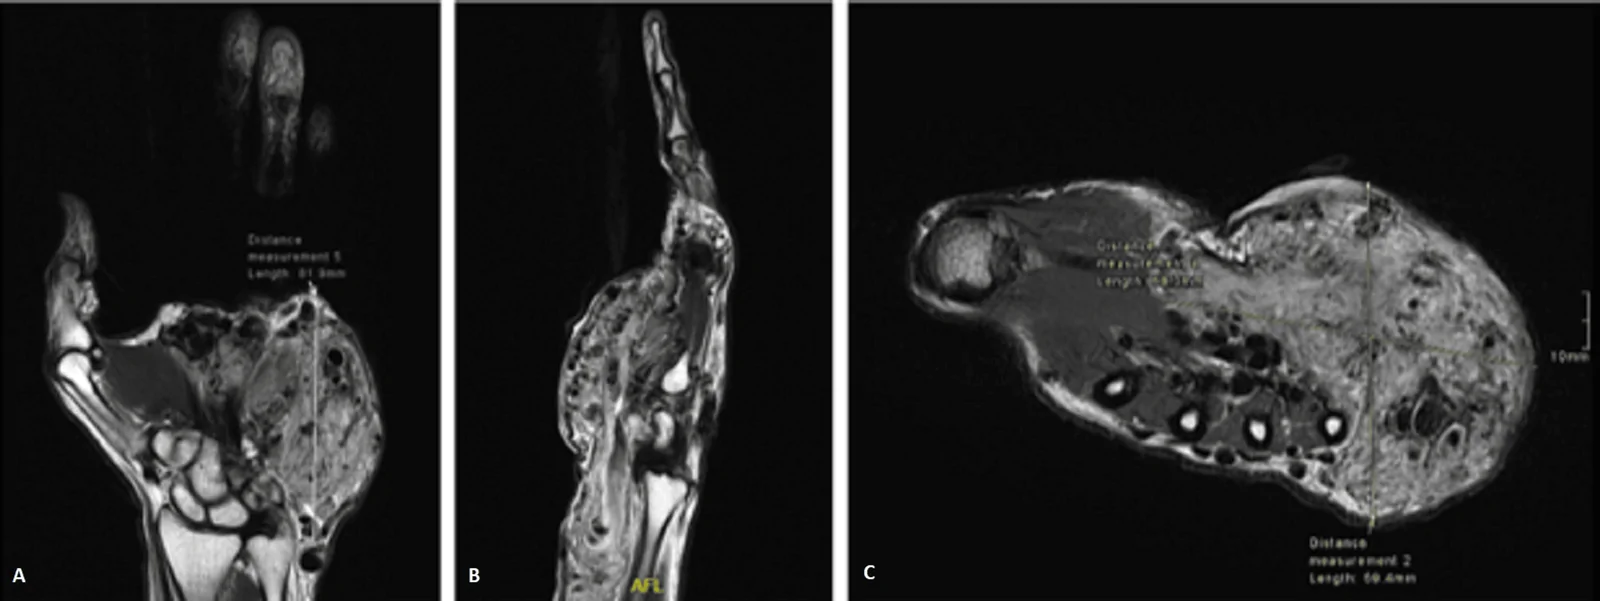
Left hand MRI with coronal (A), sagittal (B) and axial planes (C) Large complex high-flow arteriovenous malformation (AVM) of the left hand involving the volar/ulnar subcutaneous tissues, with arterial feeders from both radial and ulnar arteries and early venous drainage. These vessels extend from the distal forearm into the malformation and continue distally to the level of the proximal digits. The overall malformation and soft tissue complex measures approximately 8 x 7 x 6 cm (CC x TS x AP dimensions).
